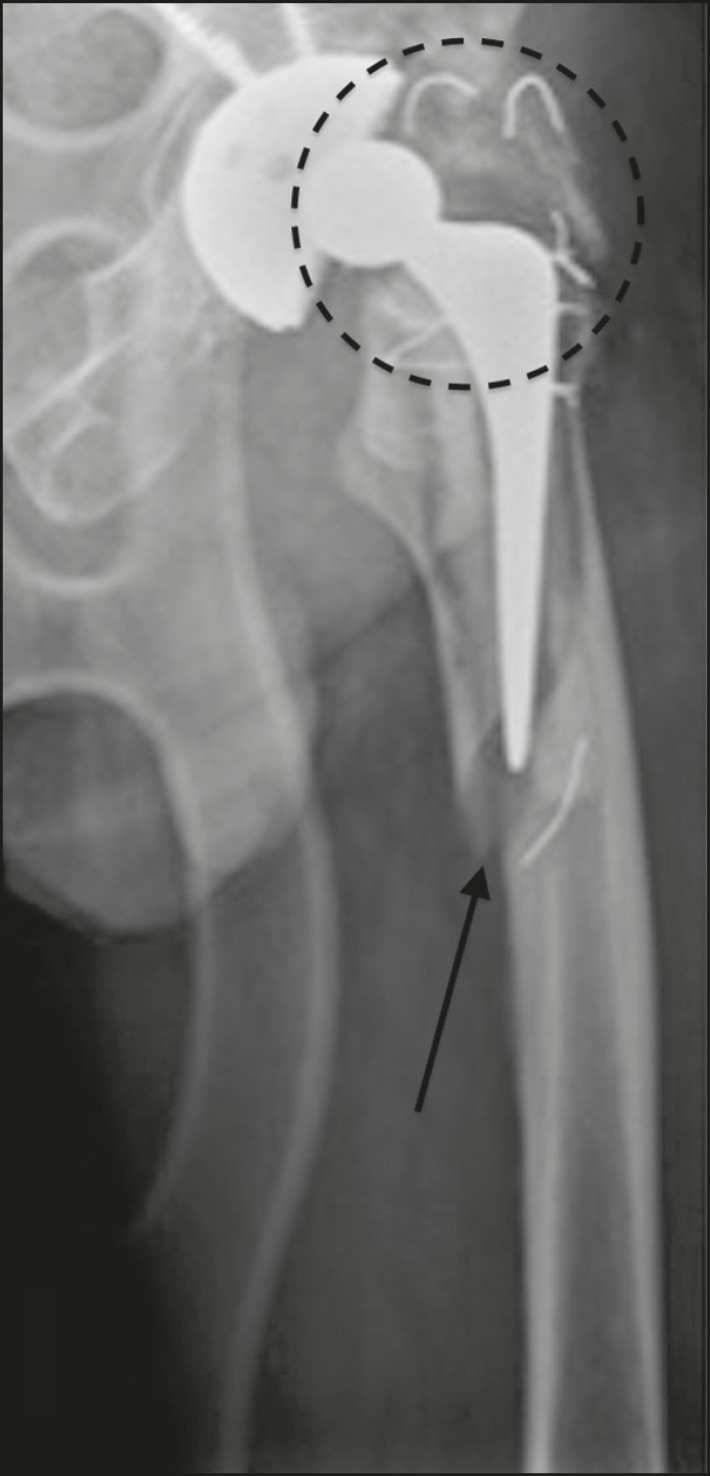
Anteroposterior X-ray of the left hip identifying periprosthetic fracture near the distal femoral stem (arrow), with signs of prosthesis instability (Vancouver subtype B2). Fracture of the greater trochanter with breaks in the metal bands (dotted circle).
